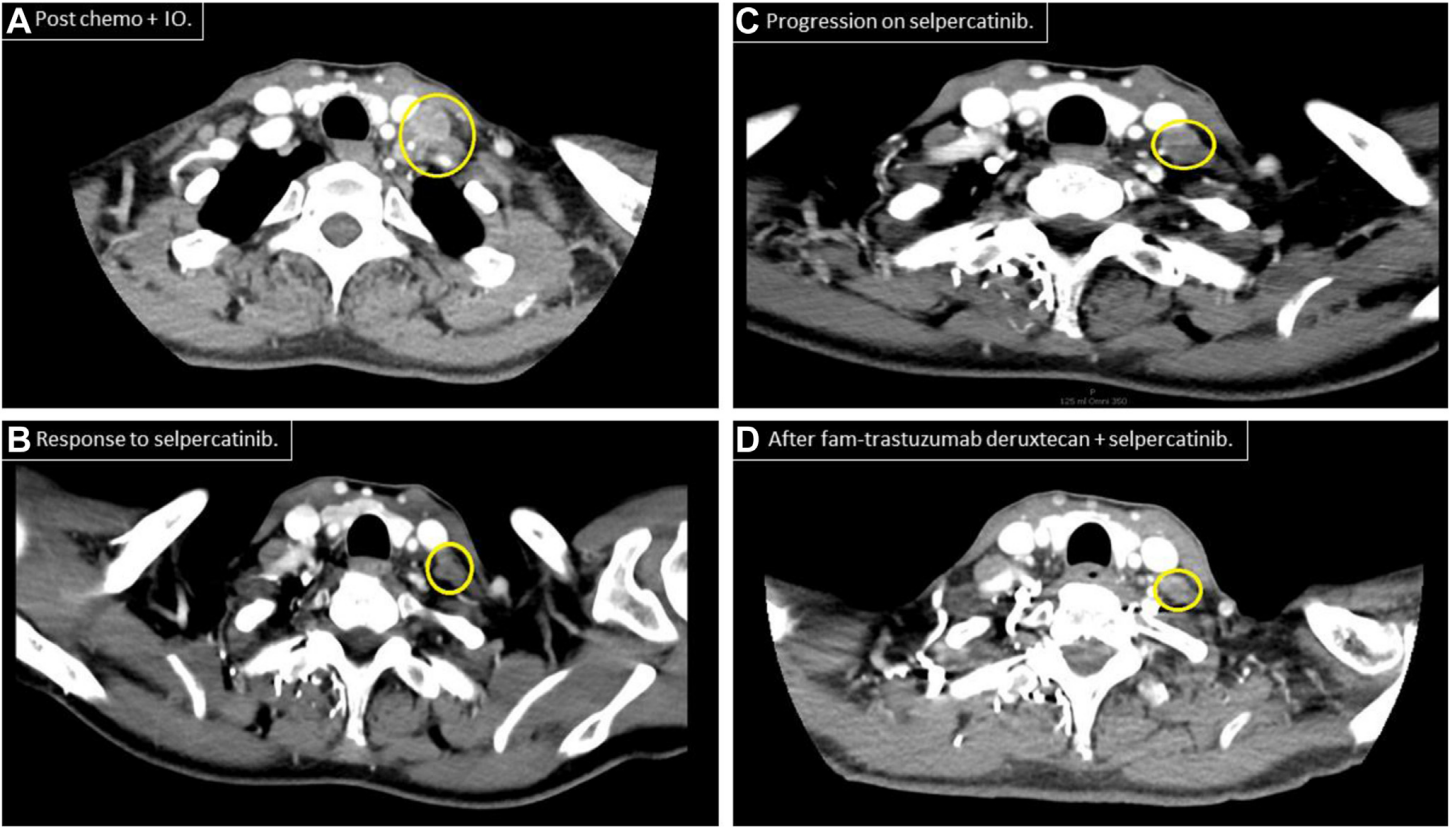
CT scans of the neck with the relevant lymph nodes circled. Panel *A* was before initiation of selpercatinib (1.6 cm in size), panel *B* with decrease in size after 15 months of selpercatinib, panel *C* at time of progression in the neck, and panel *D* after SBRT and then eight cycles of fam-trastuzumab deruxtecan plus selpercatinib. Chemo, chemotherapy; CT, computed tomography; IO, immune-oncology; SBRT, stereotactic body radiation therapy.
